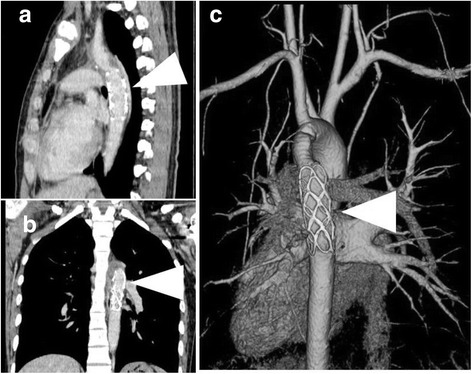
Postoperative contrast-enhanced computed tomography. a Horizontal dislocation. b Sagittal section. c Three-dimensional computed tomography. The arrowhead shows the stent graft
